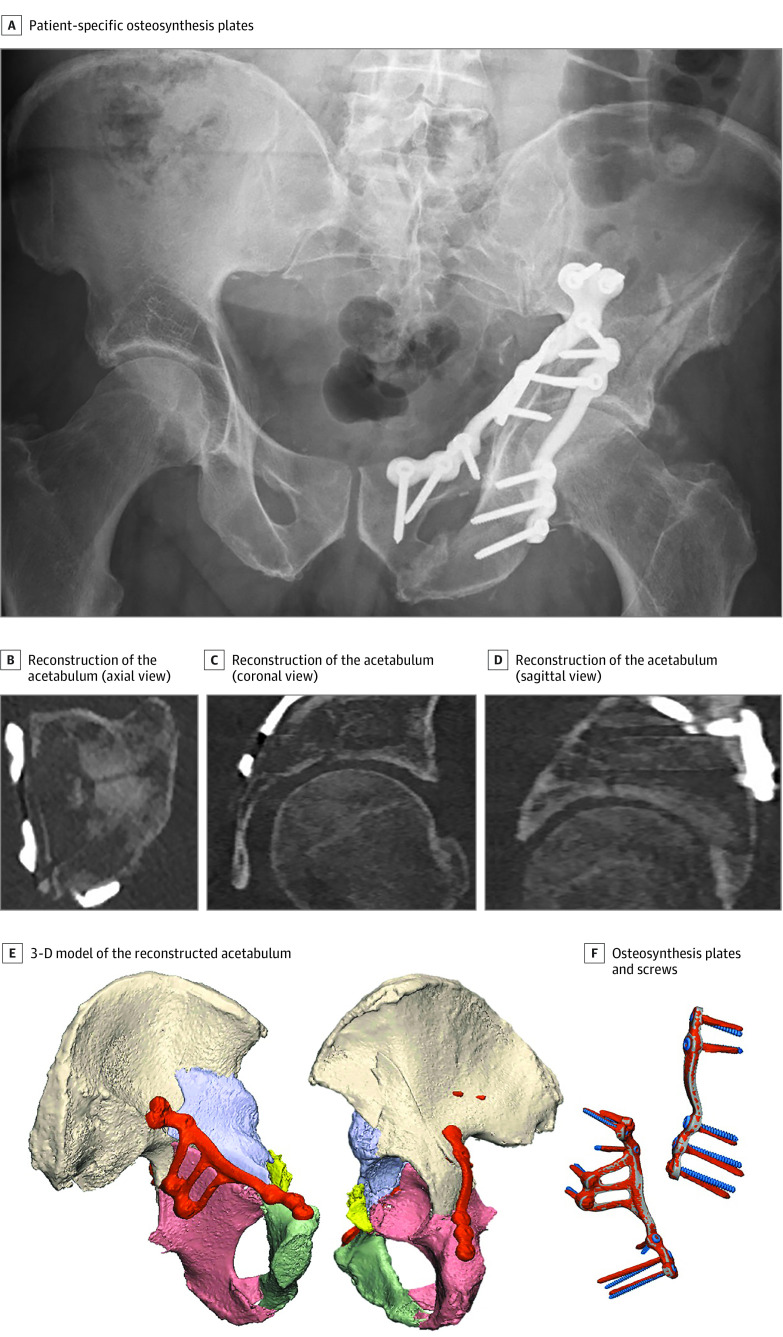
Postoperative Situation Patient-specific osteosynthesis plates were placed in patient 5 according to the preoperative plan. A postoperative computed tomography (CT) scan demonstrated good reconstruction of the fractured acetabulum. The gap was reduced from 28 mm before the operation to 5 mm after the operation. The step-off was reduced from 15 mm before the operation to 2 mm after the operation. A 3-dimensional (3-D) model, generated from the postoperative CT data, demonstrated an accurate surgical reconstruction of the fractured acetabulum (bottom left and middle). The osteosynthesis plates and screws, retrieved from the postoperative CT scan (orange), were digitally matched with the preoperative planning (gray and blue, F). The matched osteosynthesis plates demonstrated that all screws had been inserted accurately with only a median deviation of 6.8 degrees between the preoperative planning and the actual execution. The patient recovered uneventfully and returned to work after 6 months of rehabilitation. At the 1-year follow-up, the patient had no complaints about the hip, with no pain or physical impairment.
